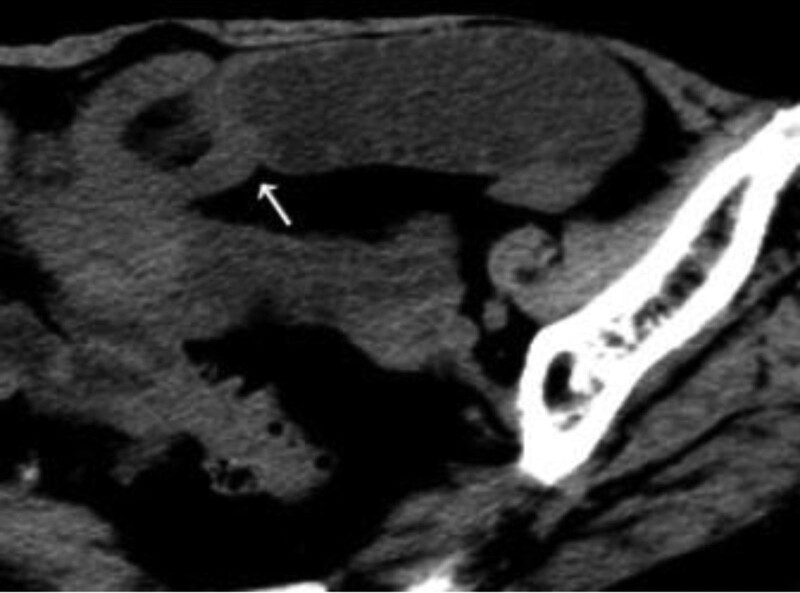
An abdominal computed tomography of small bowl intussusception showed “cuppy sign” (white arrow) presenting with obstruction.
